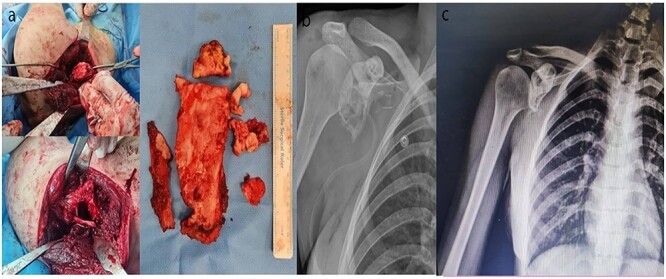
(a) Wide resection of the tumor through Judet approach; (b) post-op X-ray after 6 weeks and (c) post-op X-ray after 18 months.
